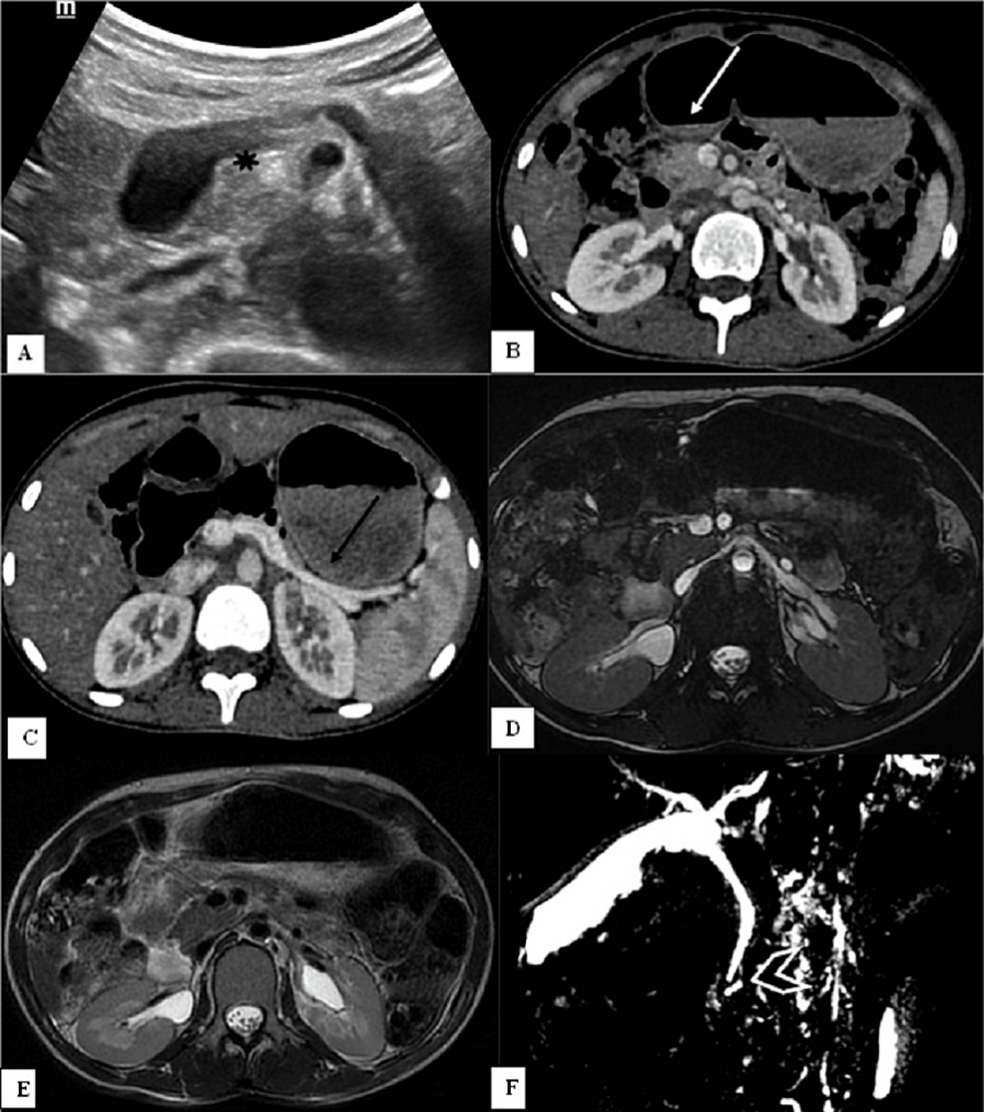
Complete dorsal agenesis of the pancreas. Transabdominal ultrasonography of a 19-year-old girl showing visualization of only the uncinate process and part of the head of the pancreas (A, black star). On the axial section, contrast-enhanced computed tomography scan images show the head of the pancreas (B, white arrow) but the neck, body, and tail of the pancreas are not visualized and potential space is filled with stomach and intestinal loops (C, black arrow). Magnetic resonance imaging axial fast imaging employing steady-state acquisition (FIESTA) (D) and axial T2-weighted image (E) showing the absence of body and tail of pancreas and computed tomography equivalent of the dependent stomach and dependent intestine sign. Magnetic resonance cholangiopancreatography (MRCP) (F) demonstrates a short major pancreatic duct, i.e., the duct of Wirsung (chevron arrow) and dorsal duct is not visualized.
